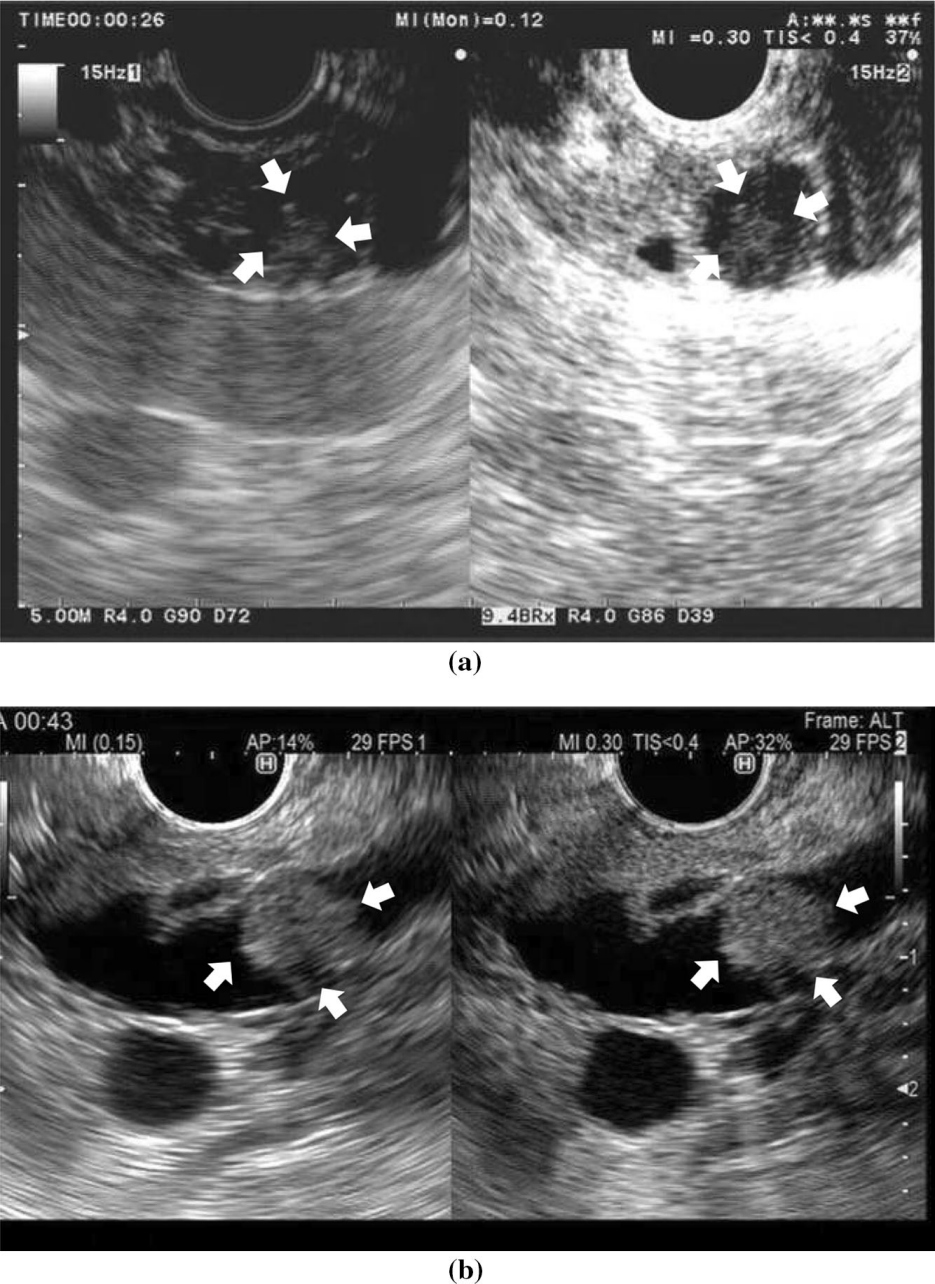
a A case of intraductal papillary mucinous neoplasm (IPMN) with mucous clot. Conventional EUS shows echogenic mural lesions (arrowheads) in a cyst cavity (left). Contrast-enhanced harmonic endoscopic ultrasonography (CH-EUS) shows no vascularity in the mural lesion (arrowheads) (right). b A case of IPMN with mural nodule. Conventional EUS shows echogenic mural lesions (arrowheads) in a cyst cavity (left). CH-EUS shows vascularity in the mural lesion (arrowheads) (right)
